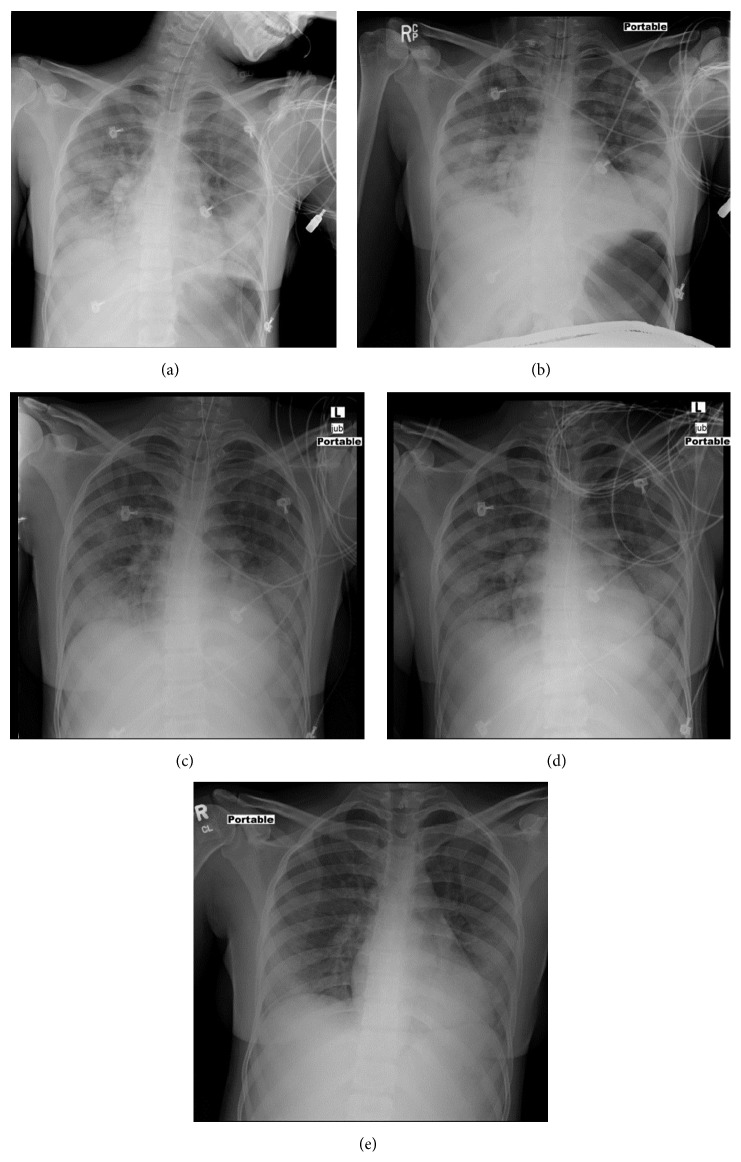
(a)–(d) Endotracheal tube is noted with the tip ending at the satisfactory position. An enteric tube has been placed. Diffuse bilateral hazy opacification is again seen, which gradually improved in sequential (a)–(d) chest radiographs. (e) Chest radiograph showing marked improvement in previously seen bilateral hazy opacities after extubation.
